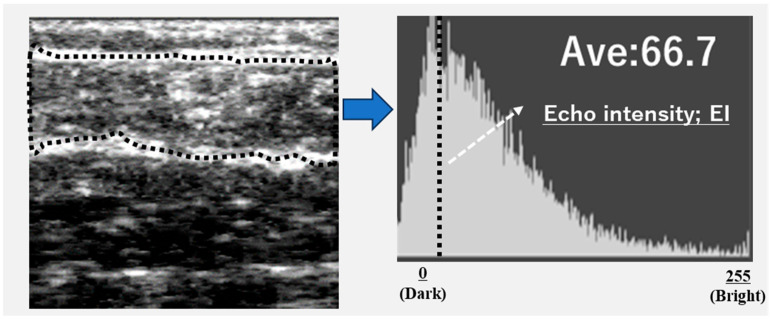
Calculation of echo intensity from the ultrasonographic image.
